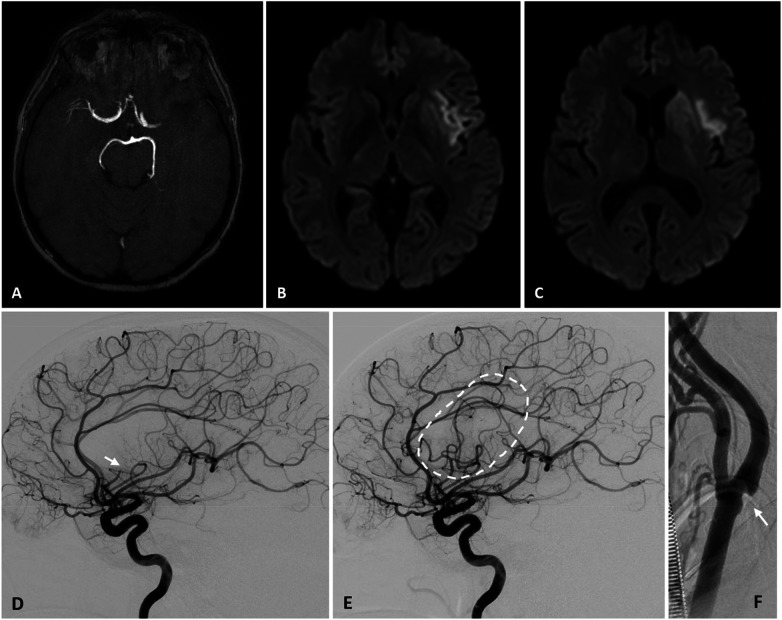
A 54-year-old woman with no cardiovascular risk factors presented with sudden-onset right-sided hemiplegia and aphasia. Emergency cerebral MR angiography revealed an occlusion of the M1 segment of the left middle cerebral artery (A), resulting in a deep MCA territory infarction (B, C). Intravenous thrombolysis was initiated during transfer from a peripheral hospital to the comprehensive stroke centre for thrombectomy. On arrival, initial angiographic assessment (D) demonstrated an occlusion of a left M2 branch (arrow). (E) Post-thrombectomy angiogram following a single pass with a stent retriever showed successful recanalisation and reperfusion of the downstream territory (dashed line). Final angiographic images at the left carotid bifurcation (F) revealed a carotid web (arrow). After excluding haemorrhagic transformation on CT at 24 h post-stroke, antiplatelet therapy with aspirin 160 mg/day was initiated for secondary prevention.
